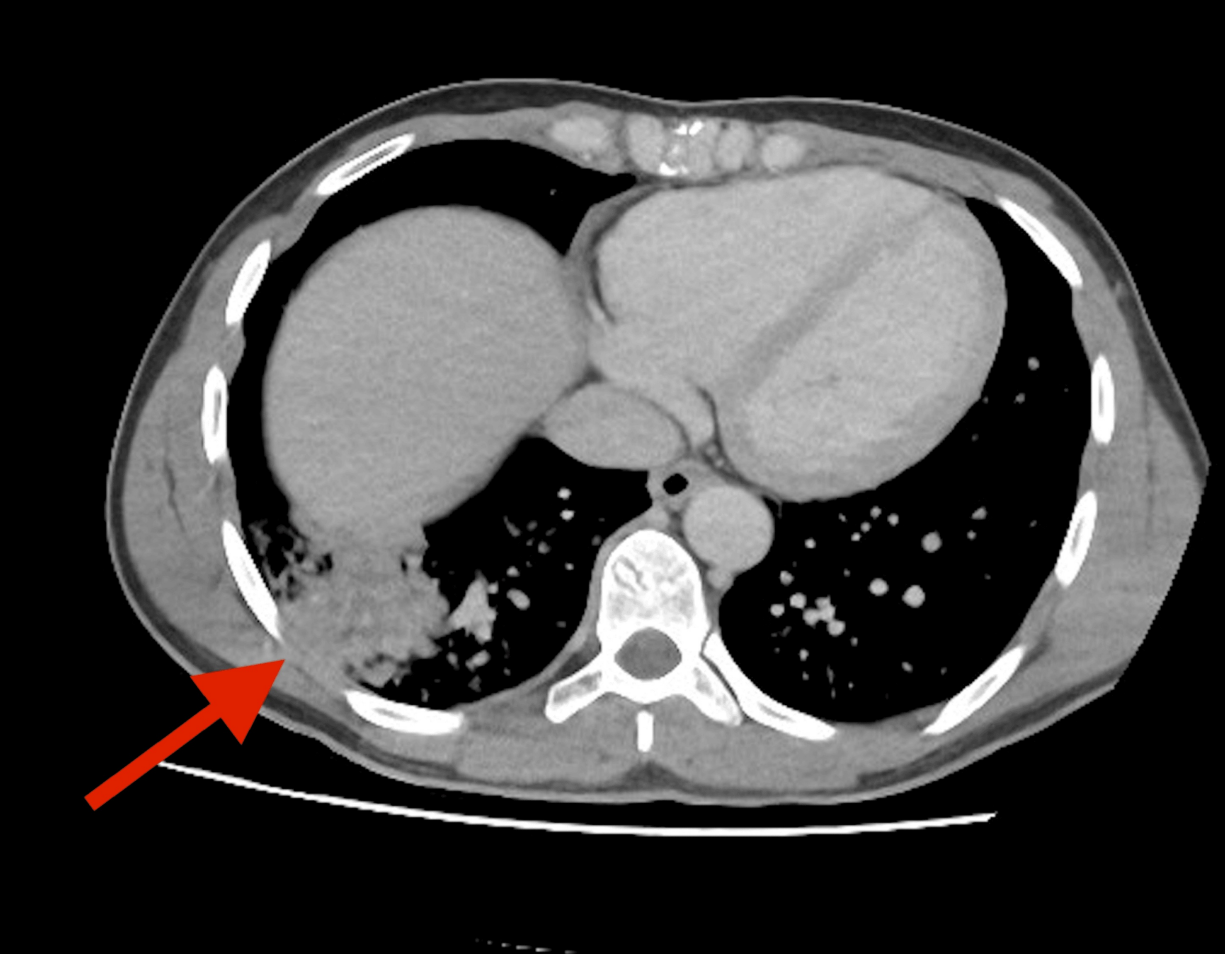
CT abdomen pelvis (axial plane) showing right lower lung consolidation
